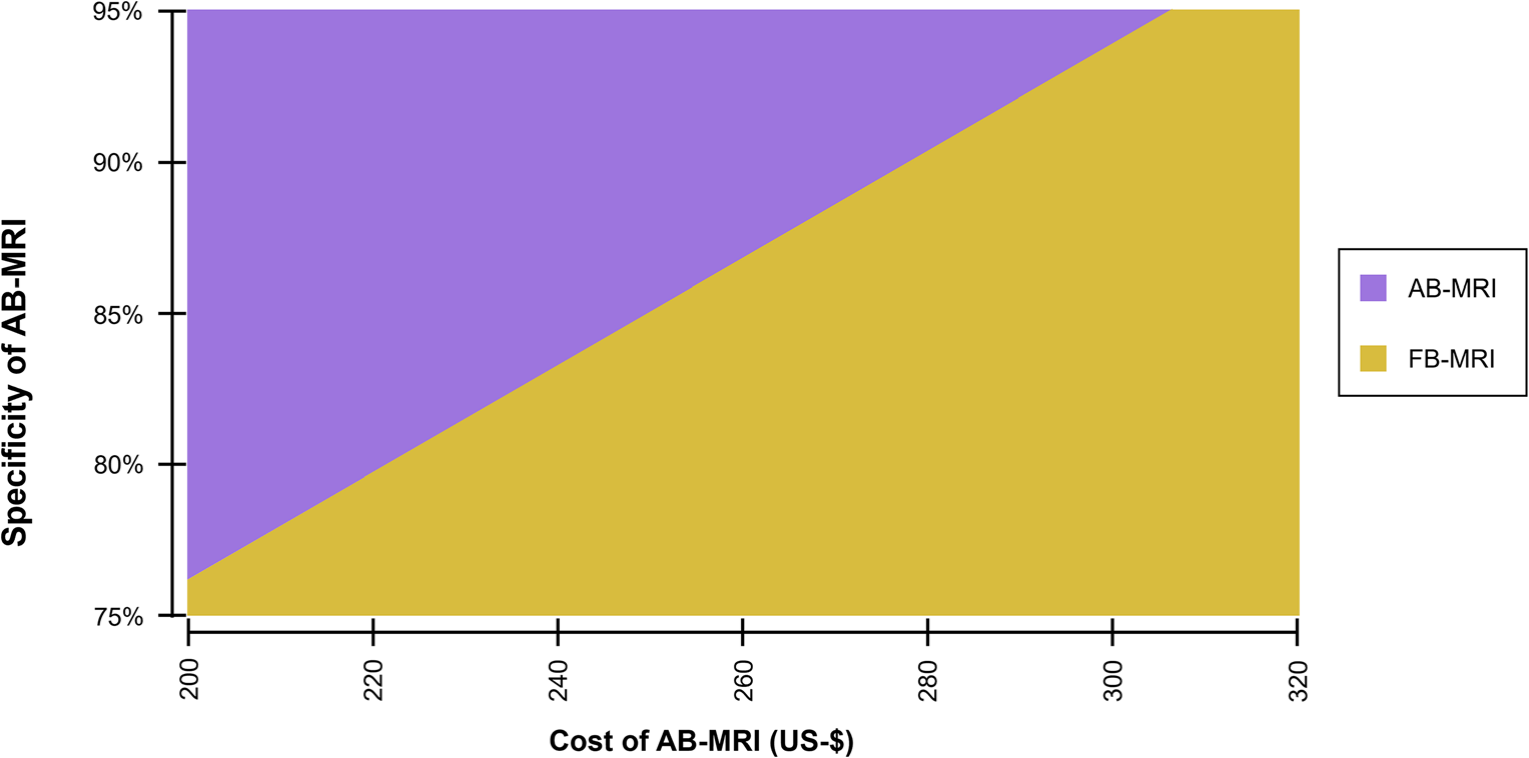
Two-way sensitivity analysis of the net monetary benefit for varying cost per examination and varying specificity of abbreviated breast MRI (AB-MRI). The color-coded area indicates the dominant strategy at a given cost and specificity of AB-MRI. The smaller the cost and the higher the specificity of AB-MRI, the more likely it ought to be preferred to full protocol breast MRI (FB-MRI). AB-MRI, abbreviated breast magnetic resonance imaging; FB-MRI, full protocol breast magnetic resonance mammography
